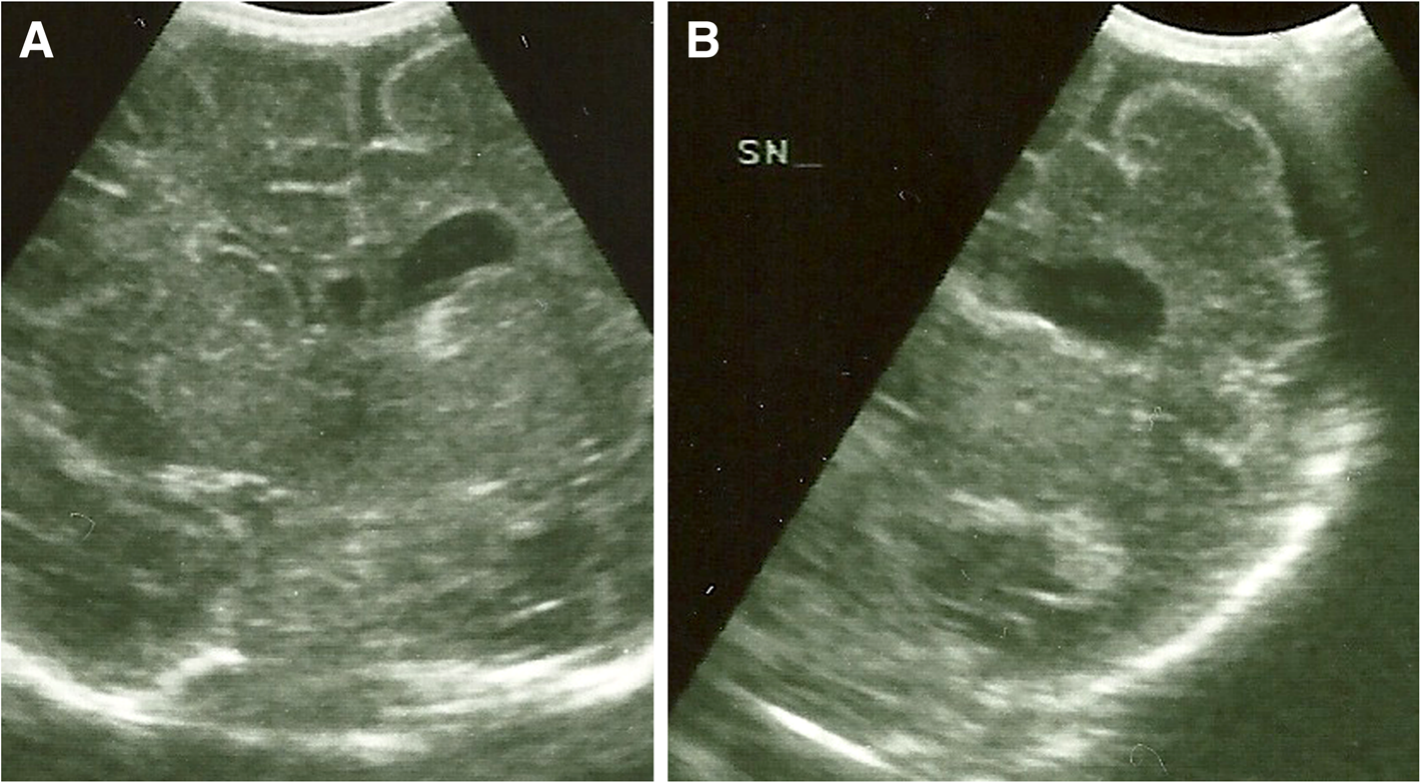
Neonatal cerebral ultrasonographic coronal images. A Left ventriculomegaly, B Left hemisphere atrophy with enlarged subarachnoid spaces.
